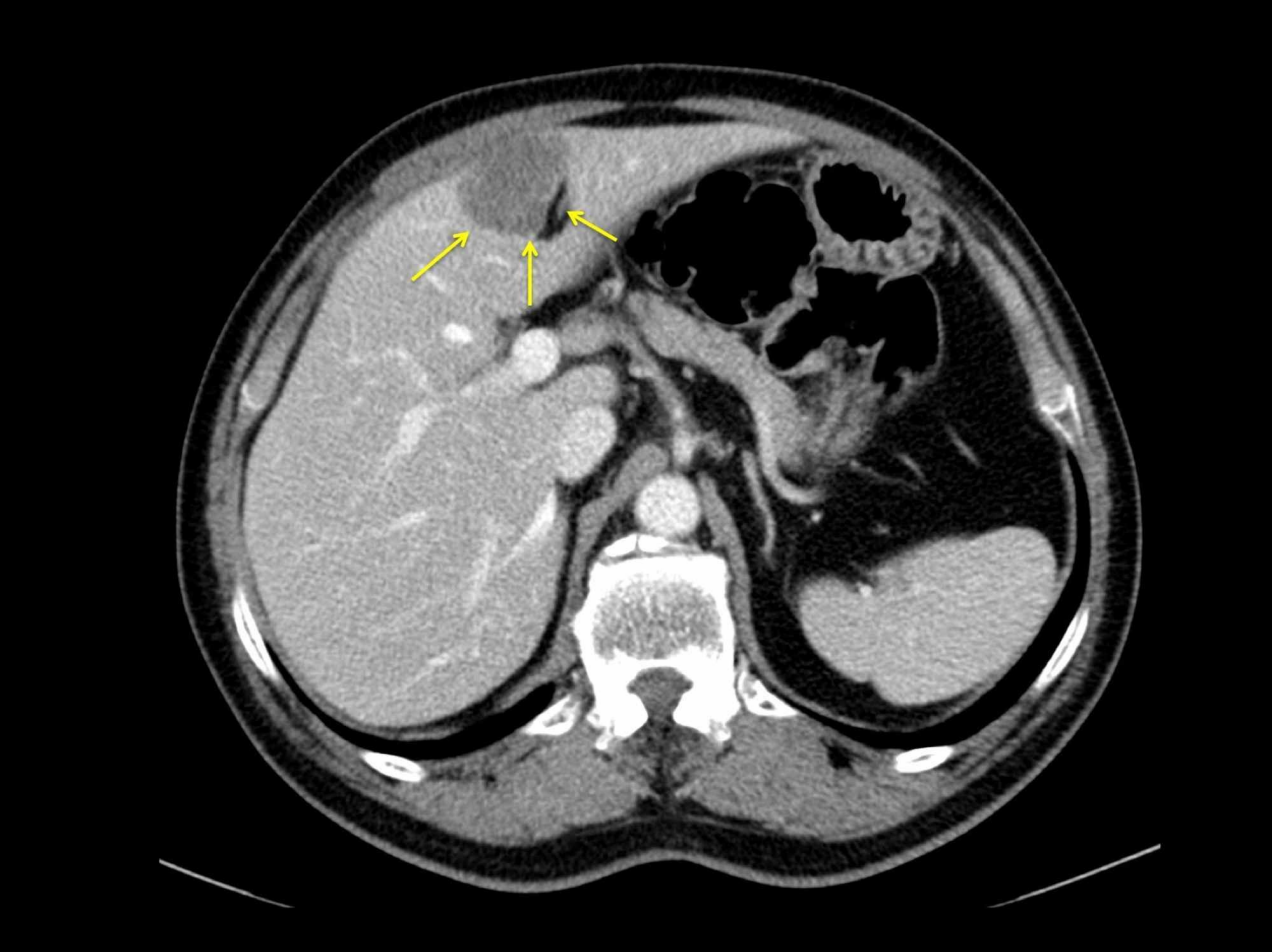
A staging computed tomography scan revealed a 4 cm metastatic deposit in the left lateral segment of the liver
